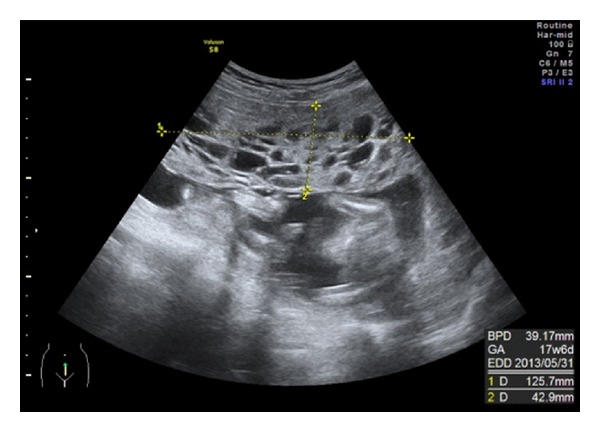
An ultrasonography showing a large multicystic placenta.
